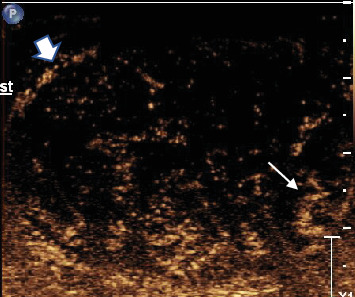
Contrast-enhanced ultrasound image of the lesion using perflutren microbubbles in the late arterial phase. The bubbles within intralesional vessels are bright echoes, whereas avascular areas remain dark or anechoic and represent cystic spaces or necrotic areas. The typical enhancing “twisted vessels” (thin long arrow) are identified within the tumor. The peripheral rim enhancement (short thick arrow) is also appreciated. Early wash-in and early wash-out of contrast were observed.
